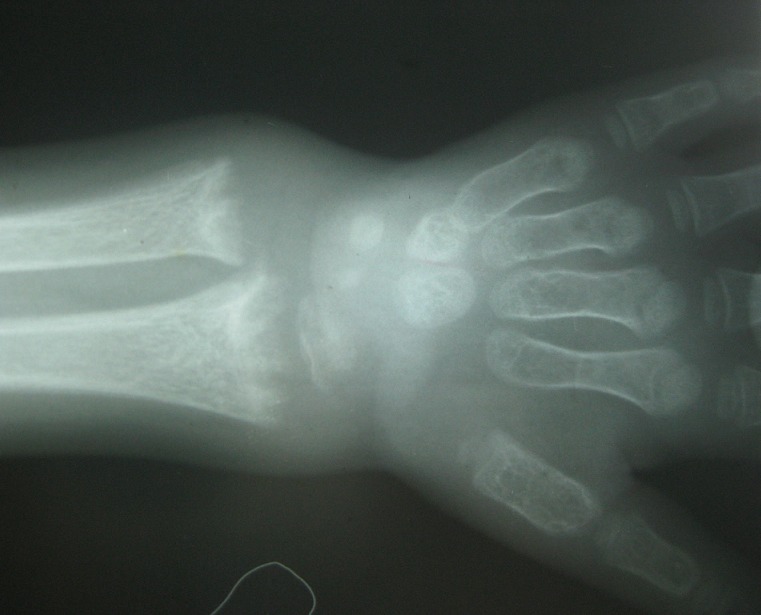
Radiographie du poignet montrant un élargissement en cupule des métaphyses dont les limites sont floues. Déminéralisation osseuse
